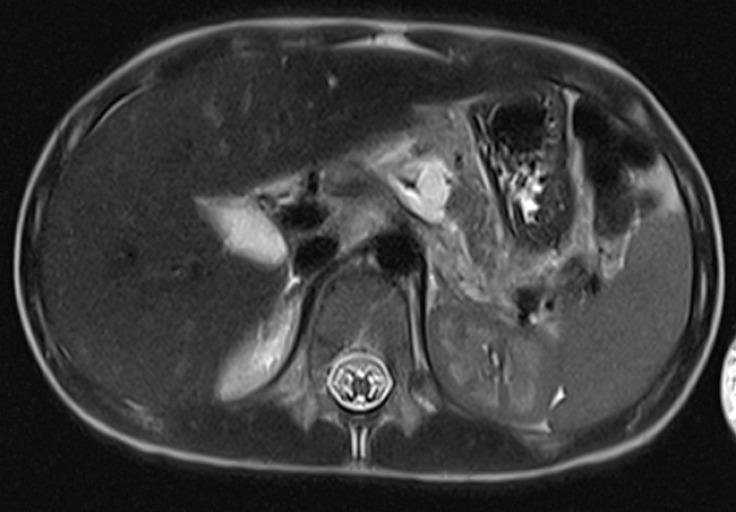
Magnetic resonance imaging (MRI) of the abdomen demonstrating pancreatic duct fracture draining into the fluid collection in the fracture plane in the body of the pancreas.
